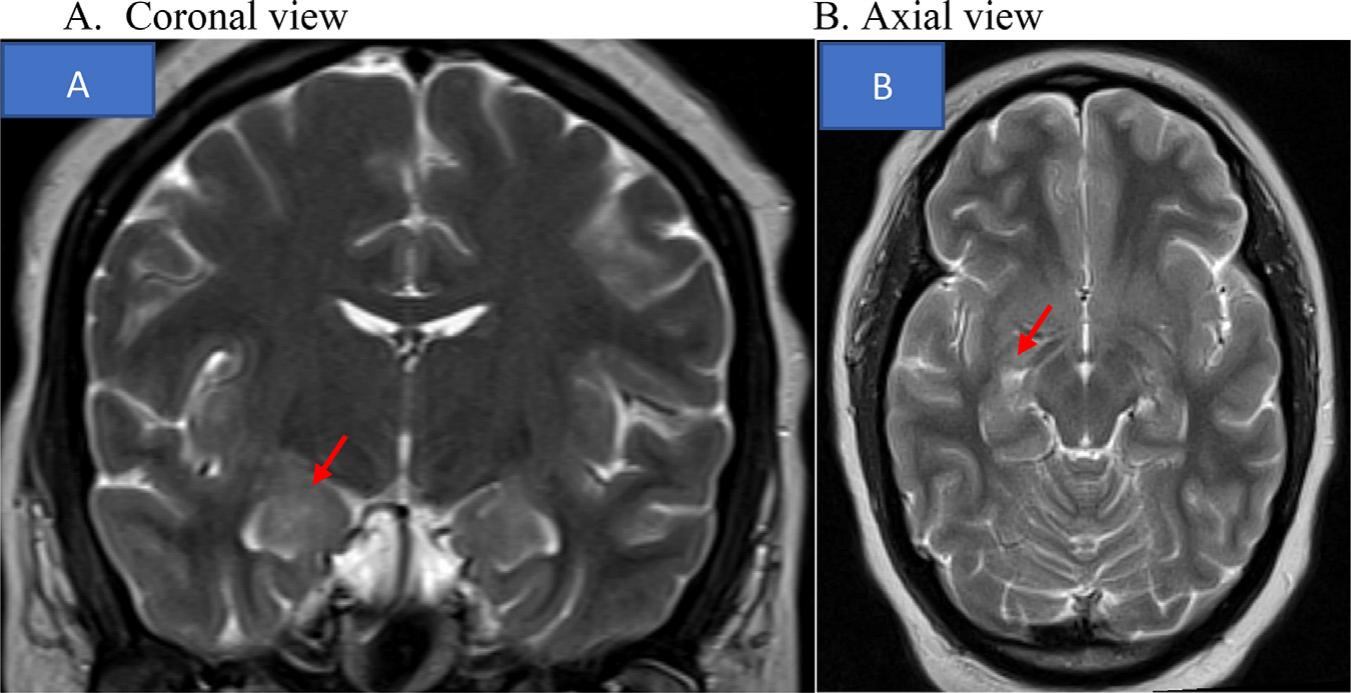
T2 Brain magnetic resonance imaging showing (A) Coronal view demonstrating increased intensity in the bilateral amygdala. (B) Hippocampal and corticospinal tracts hyperintensity.
